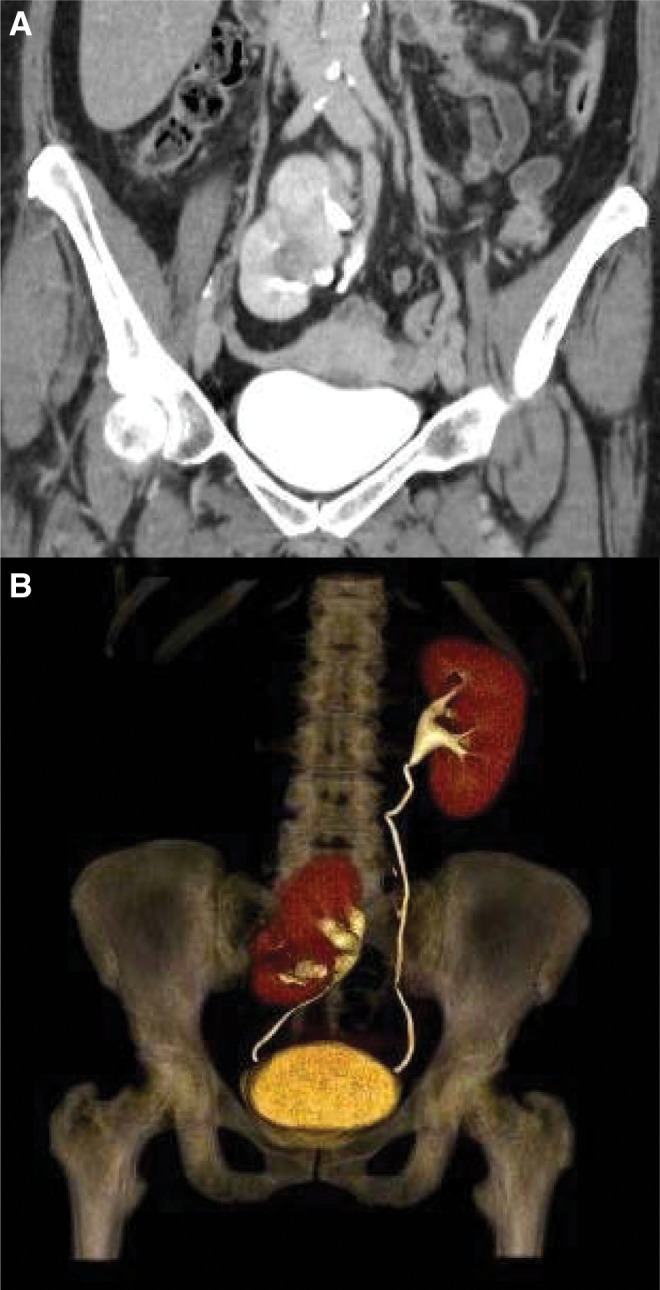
(A) Computed tomography (CT) urogram demonstrating a 3.6 × 2.0 cm heterogeneous mass originating from a right pelvic kidney, (B) CT urogram with three-dimensional (3D) reconstruction demonstrating a right pelvic kidney with associated dilation and distortion of the proximal renal collecting system.
